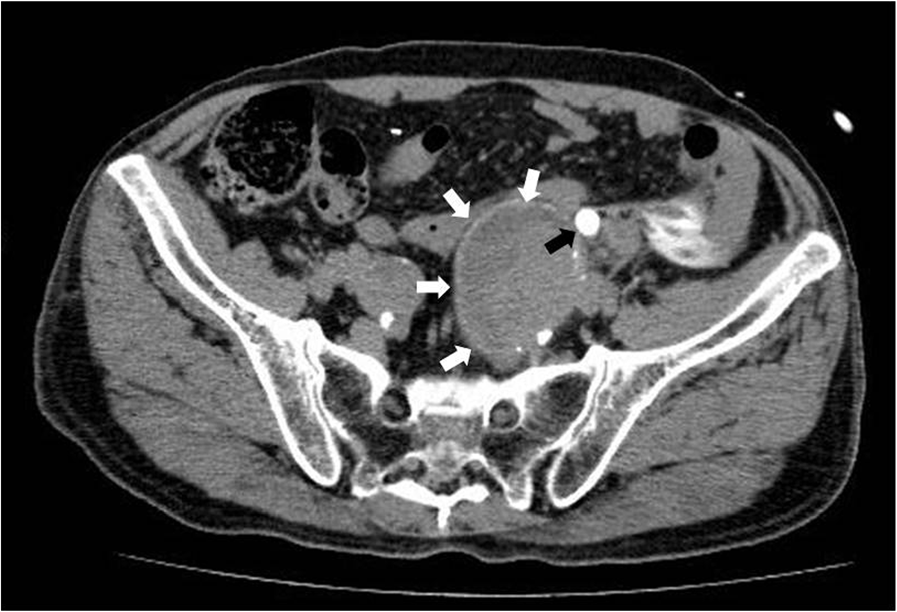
Contrast-enhanced computed tomography (CT) reveals a large left internal iliac aneurysm (white arrows). CT signs of a ruptured aneurysm are not observed. A black arrow indicates the left hydroureter
